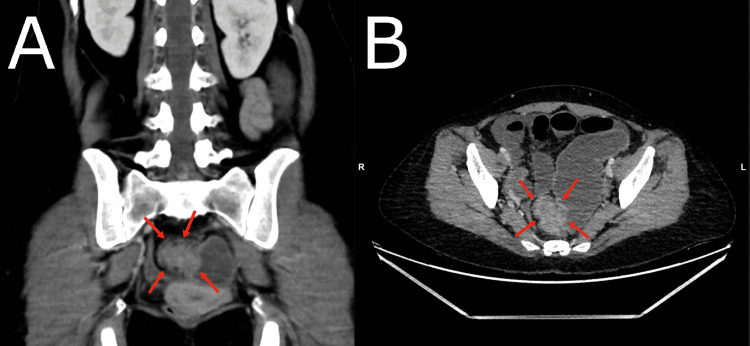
Abdominal CT scan with intravenous contrast showing a lobulated obstructing and enhancing mass lesion at the rectosigmoid junction (red arrows) in both coronal (A) and axial (B) sections. CT, computed tomography
